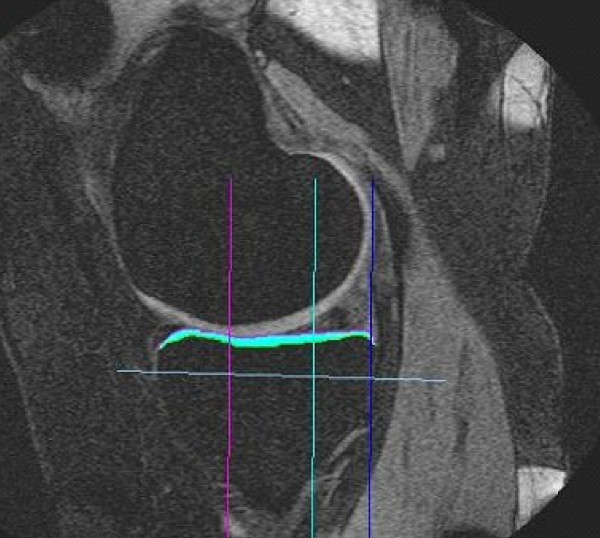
Medial tibial cartilage segmented from a single sagittal slice of an MR image acquired from a healthy knee.
